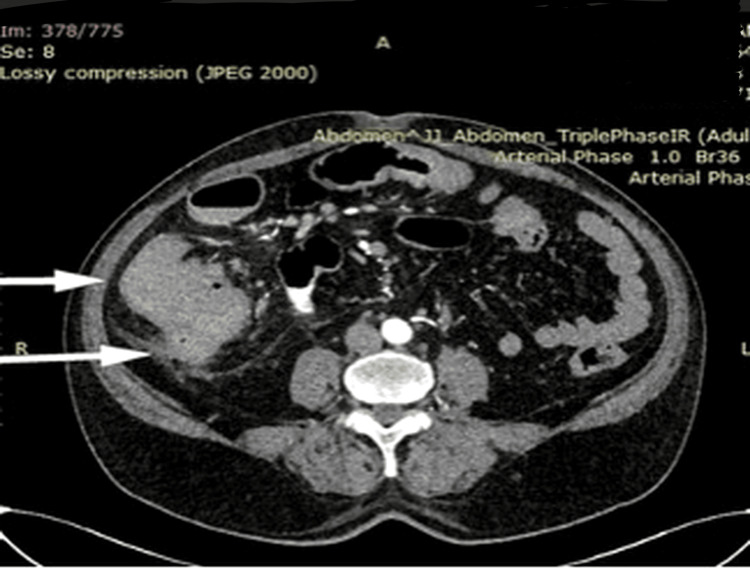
Contrast-enhanced CT abdomen (axial section) showing luminal narrowing suggestive of stricture involving the ascending colon and transverse colon.
